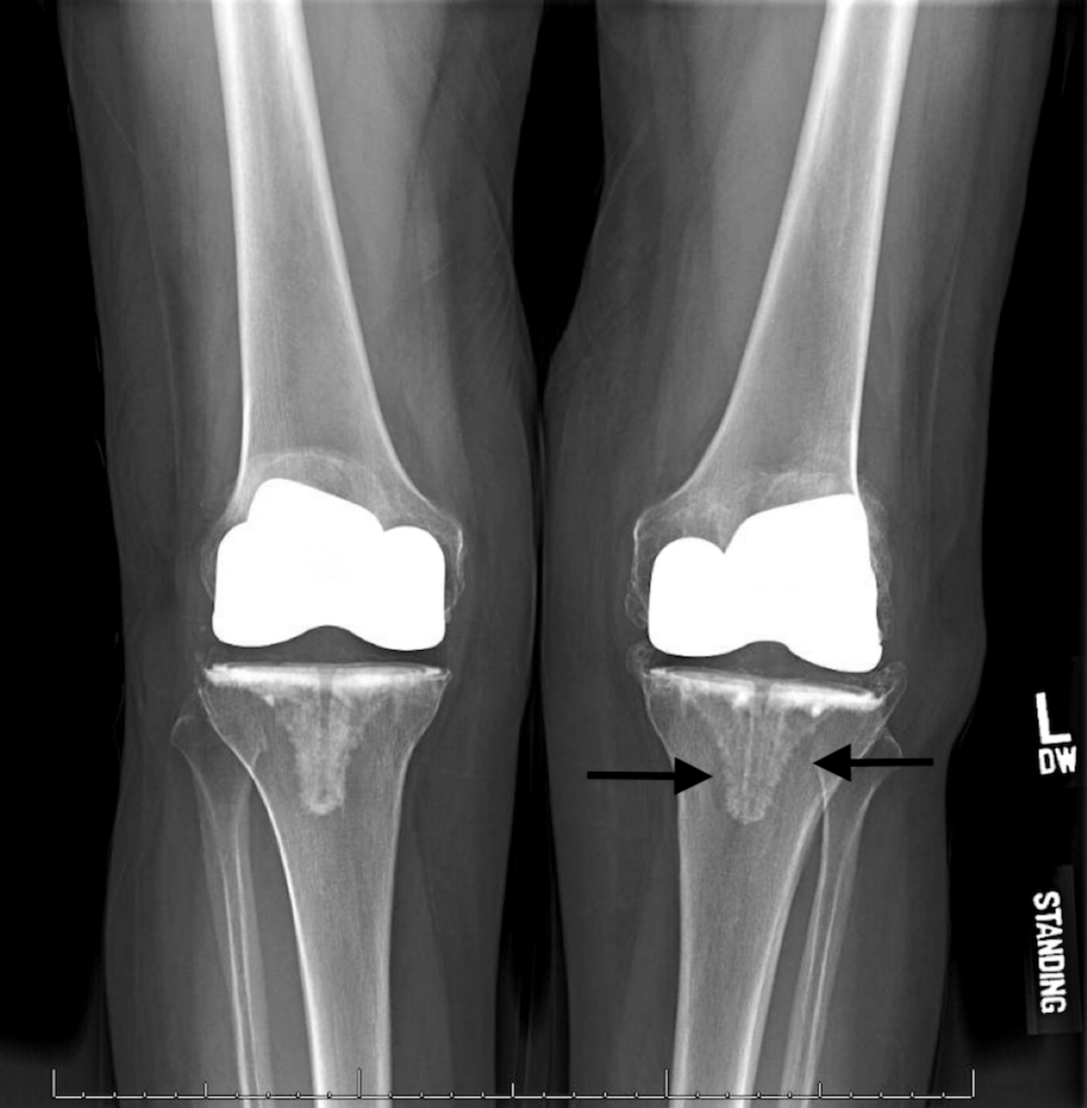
Standing radiograph of the case at initial presentation showing lysis and subsidence of left tibial component
